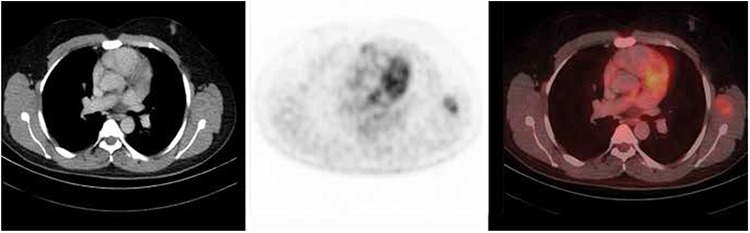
A 46-year-old-male patient with a diagnosis of myelofibrosis on bone marrow biopsy. Axial positron emission tomography-computed tomography and fusion images show hypermetabolic left subscapular soft tissue mass (SUVmax: 3.1). Biopsy of the subscapular mass revealed Non-Hodgkin’s lymphoma
